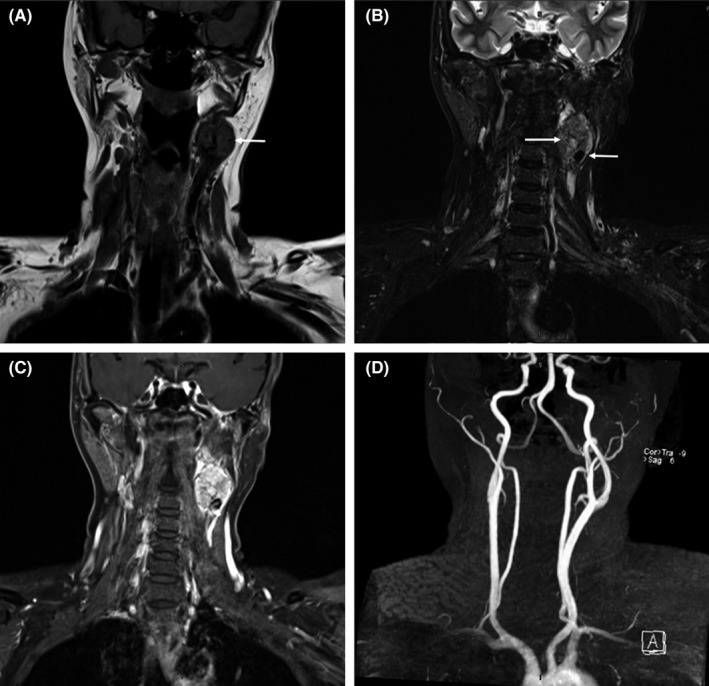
MRI of the neck, coronal view showed a heterogeneous mass with flow‐void sign and salt‐and‐pepper appearance (arrow) at left carotid bifurcation, characterized by iso‐signal intensity on T1‐weighted image A, hypersignal intensity on T2‐weighted image B, and intense gadolinium enhancement C. MRA demonstrated a prominent size of left ascending pharyngeal artery which provided feeding vessels into the mass D
